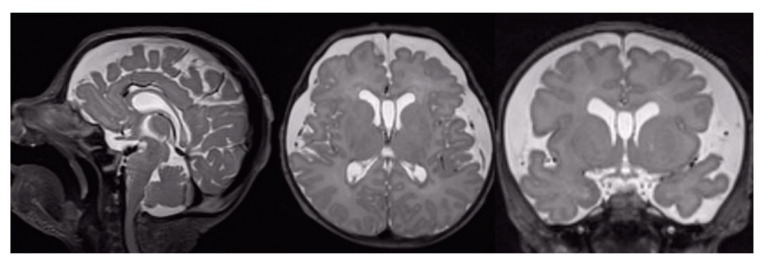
T2-weighted MRI of a CDH patient. Sagittal, axial and coronal view of the brain shows an enlargement of the SAS in the fronto-insular region bilaterally.
